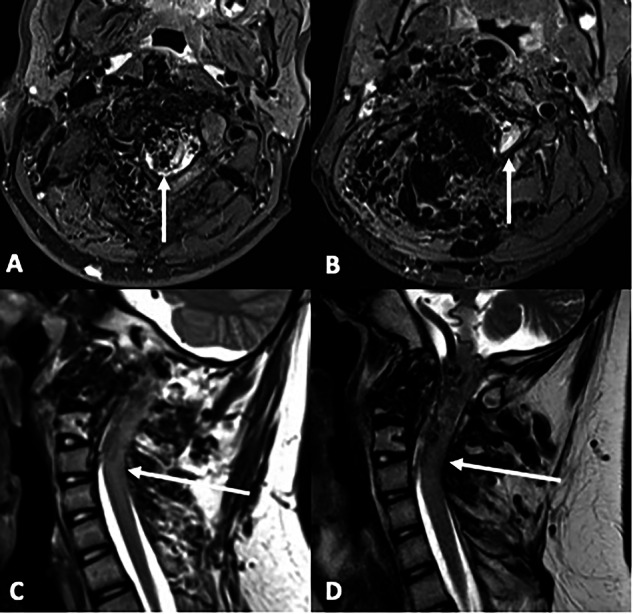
Magnetic resonance imaging in a teenage patient with cervical spinal arteriovenous metameric syndrome (SAMS) with cervical spinal cord AVM. Patient 3 in our study. A Axial fat-supressed-T2-weighted-image at the cervico-medullary junction demonstrates an intra-medullary arterio-venous malformation within the spinal cord (arrow). B More caudally, the cord is compressed (arrow) by enlarged epidural vessels (which appear black and serpiginous). C Sagittal T2-weighted-image (left para-sagittal) shows increased cervical cord signal in keeping with oedema from cord compression and congestion (arrow). D After six months of treatment with trametinib, there is a marked reduction in cord signal change/oedema, correlating with improvement in lower limb function. This patient improved from wheelchair dependent paraparesis to walking again.
